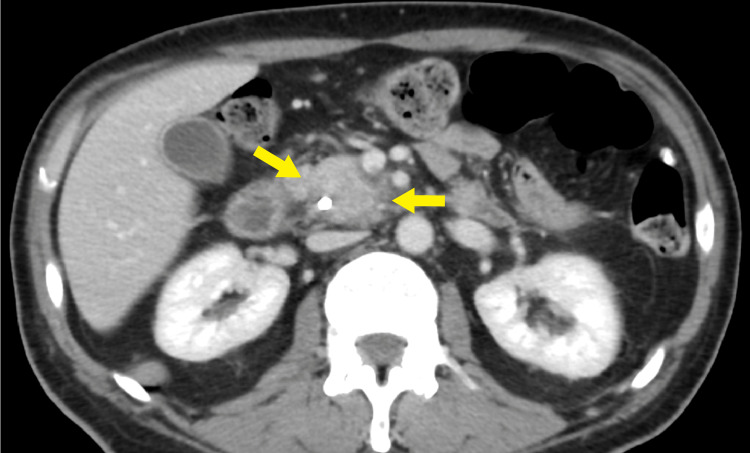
Axial CECT image showing the infiltrative hypodense pancreatic head lesion The lesion is abutting the SMA by 180 degrees. There is associated abutment and irregularity of the portal vein near the SMV, suggestive of pancreatic adenocarcinoma. The CBD stent is present. CECT: contrast-enhanced computed tomography; SMV: superior mesenteric vein; SMA: superior mesenteric artery; CBD: common bile duct
